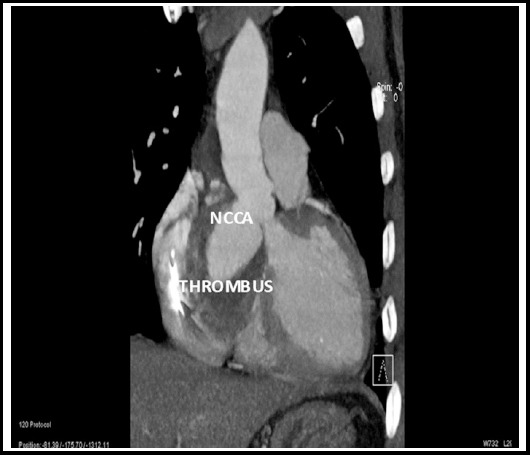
Contrast enhanced cardiac CT scan coronal view showing non-coronary cusp sinus of Valsalva aneurysm (NCCA) and thrombus.
